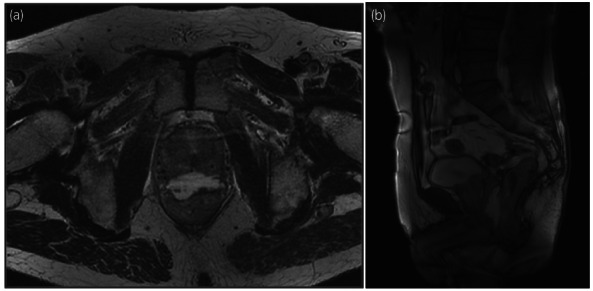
MRI after BT and the hydrogel spacer injection ––horizontal (a) and sagittal (b) views.
